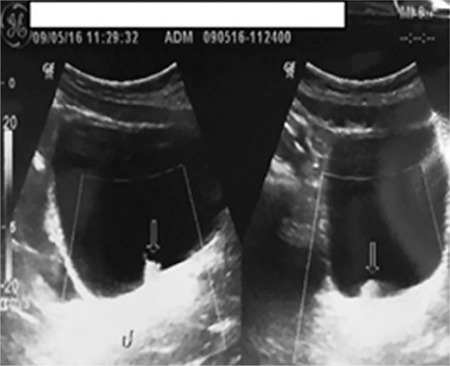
Preoperative ultrasound showing patient’s 4 bladder wall mass.
